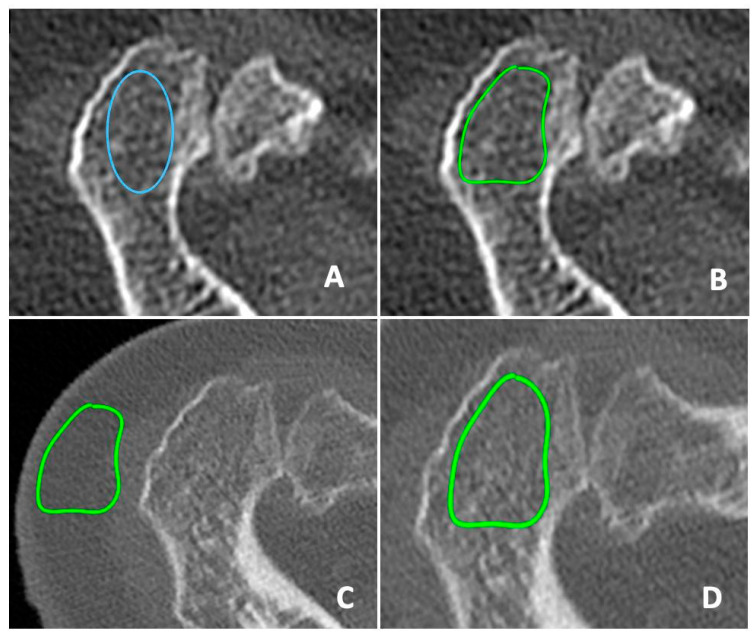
Elliptical approach (blue circle) for ROI measurement (A). Segmental approach (green circle) for ROI measurement in preoperative CT scans (B). Copy of the ROI identified in the preoperative CT scans in the postoperative CT scans to identify the same section (C). ROI measurement in the postoperative CT scans (D).
